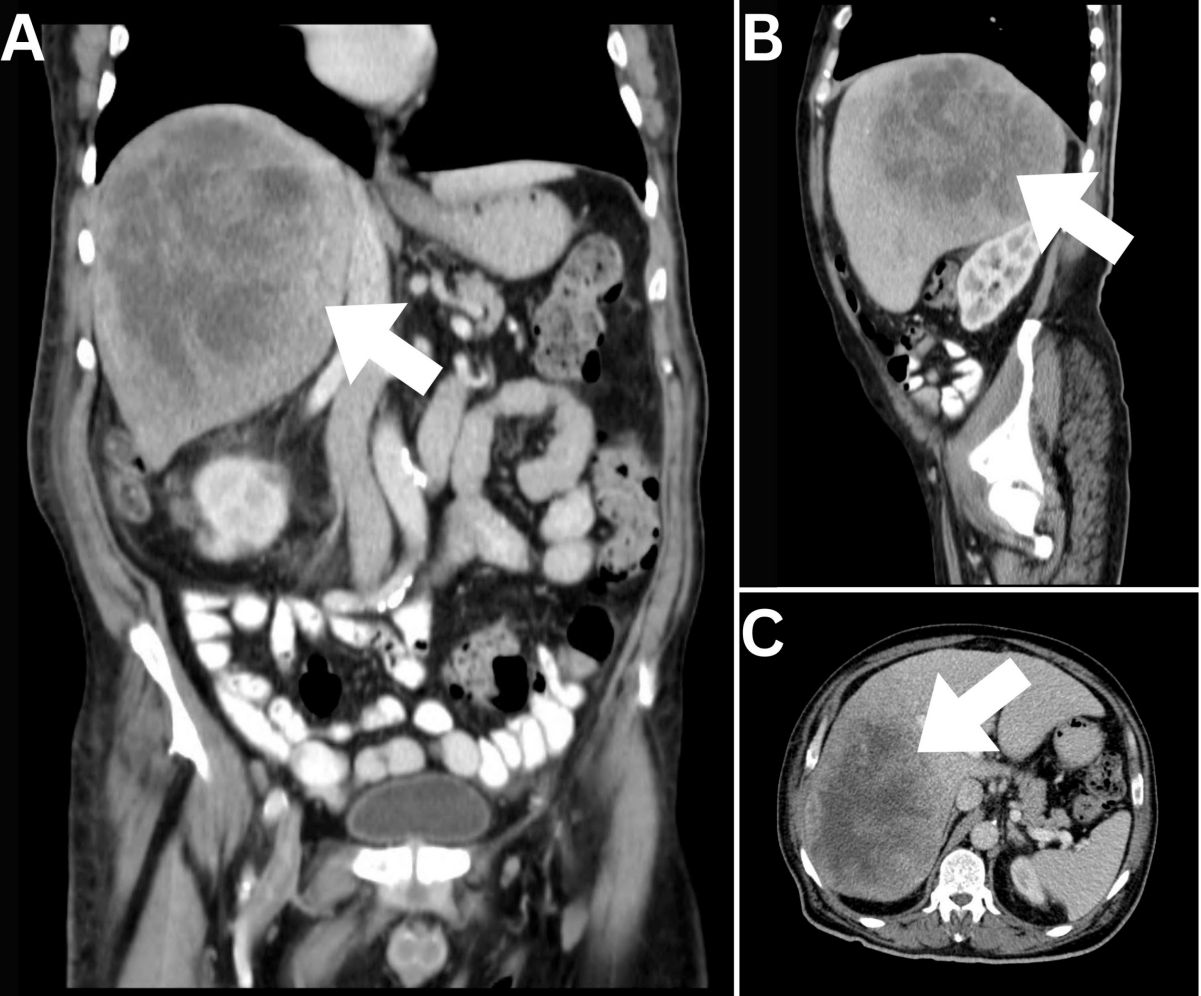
Abdominopelvic CT (A) Coronal, (B) Sagittal, and (C) Axial views of abdominopelvic CT demonstrated a large complex density mass lesion occupying a major part of the right hepatic lobe (arrows).
